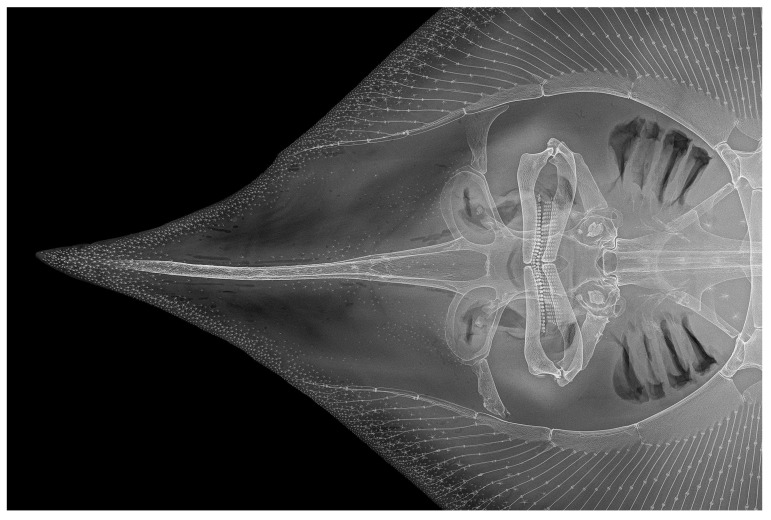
Leucoraja longirostris* n. sp., ZMH 26263, adult female paratype, 700 mm TL, radiograph of cranium and snout in dorsal view.
